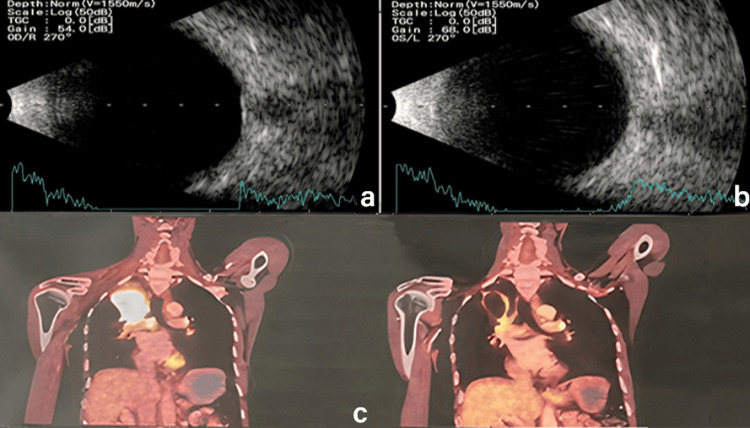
At 20-week follow-up. (a, b) B-scan ultrasound showing regression of mass lesion with a resolution of overlying subretinal fluid OU. (c) PET-CT scan showing residual lymphatic and primary pulmonary malignant pathology and regressed skeletal metastases. OU: oculus uterque; PET-CT: Positron Emission Tomography and Computed Tomography.
